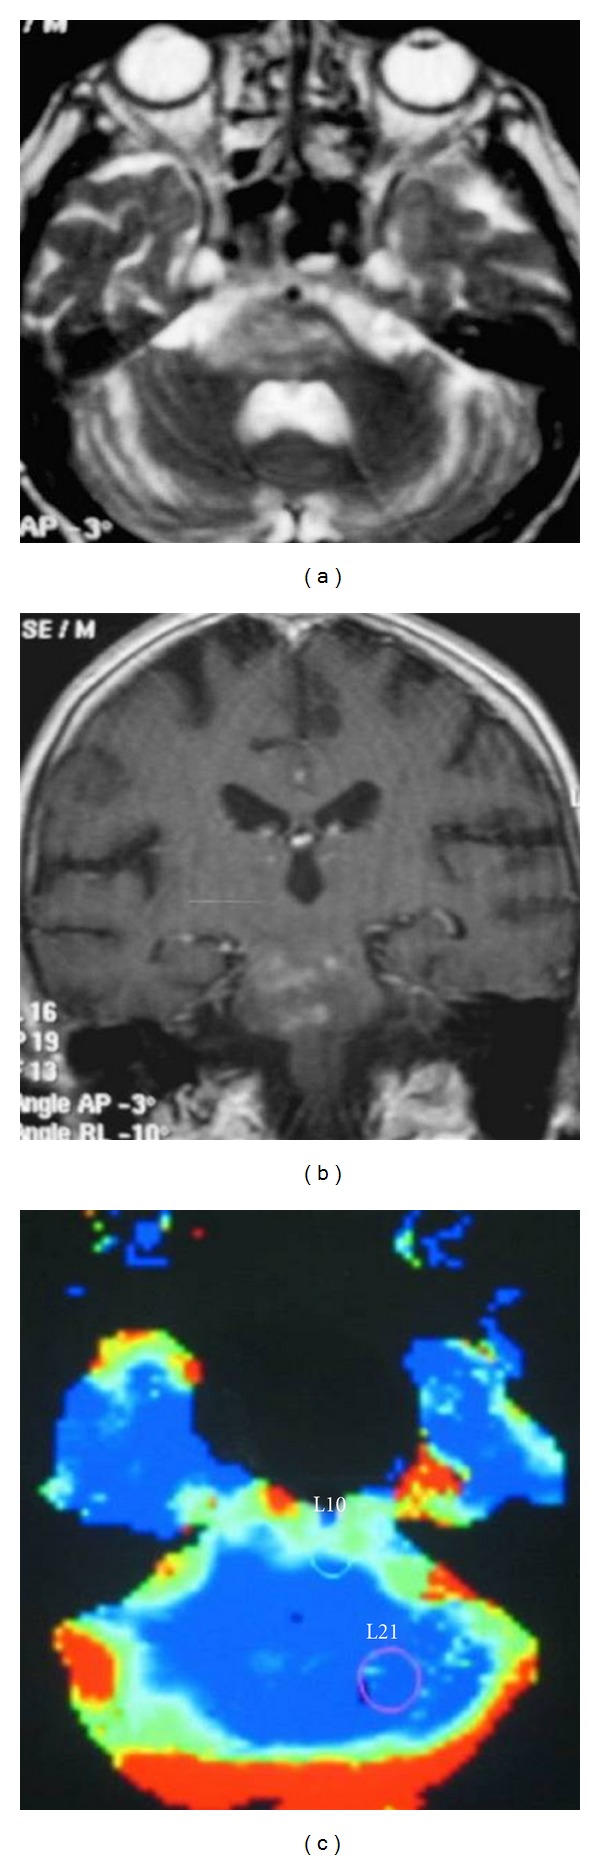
A female patient with neuro-Behcet's disease. (a) T2-weighted fast spin-echo axial MR image shows patchy hyperintensities in pons. (b) Contrast-enhanced T1-weighted echo axial MR image demonstrates nodular enhancement in pons. (c) ROIs (regions of interest) were placed into pons and cerebellar white matter in perfusion MRI.
